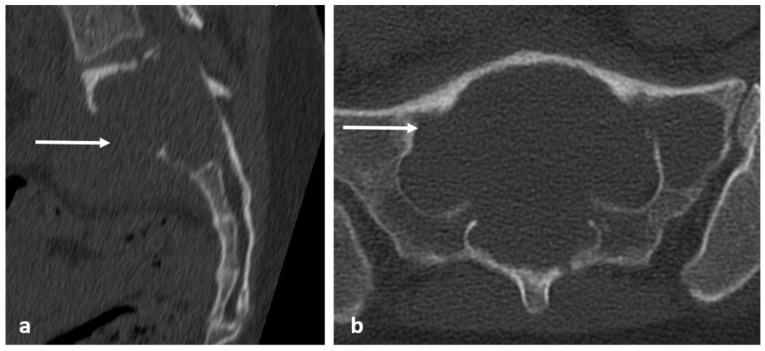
Sagittal (a) and axial (b) CT images of a sacral giant cell tumour (white arrows). The lesions typically appear lytic with thin peripheral shell of bone.
